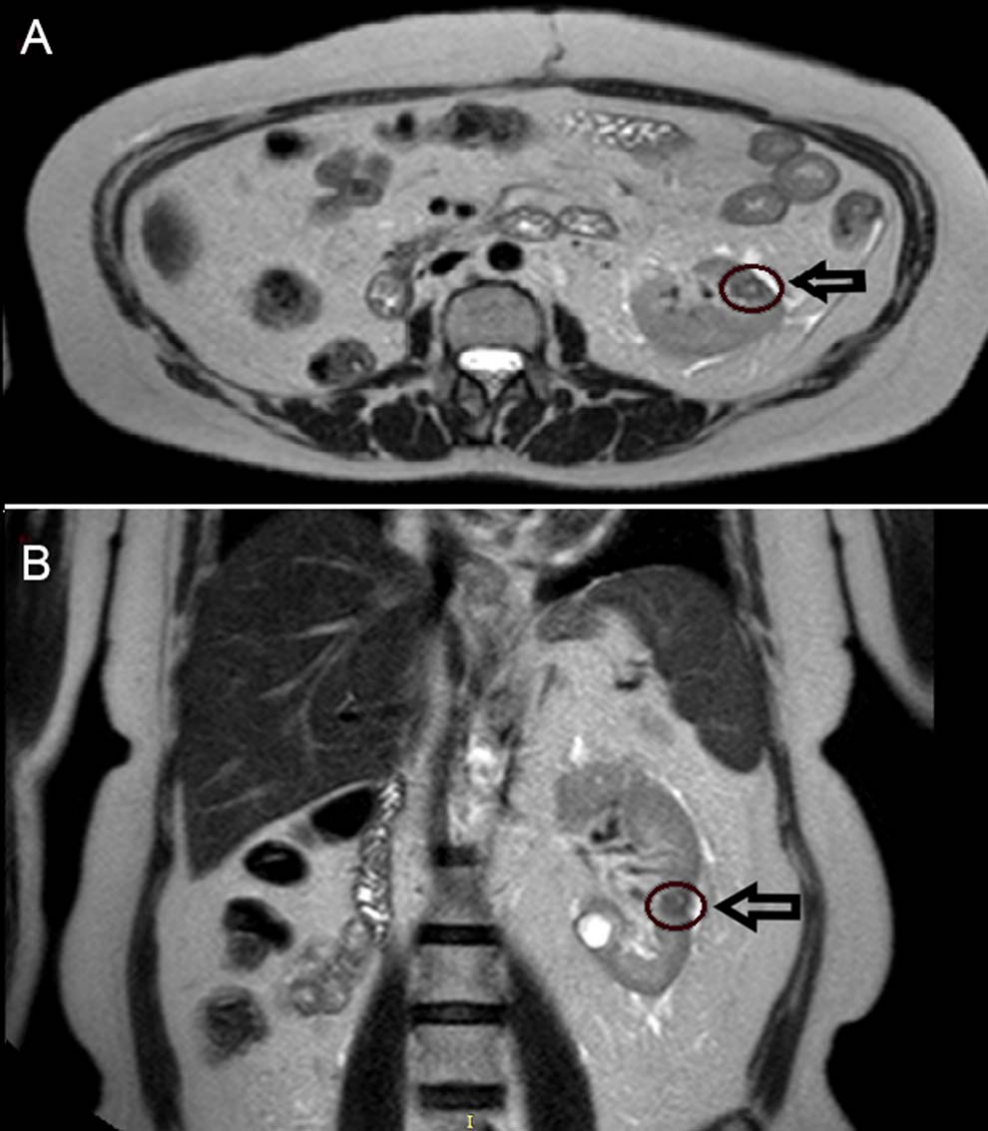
T2-weighted (A) axial and (B) coronal magnetic resonance images show the left kidney metastasis (arrows/circles).
